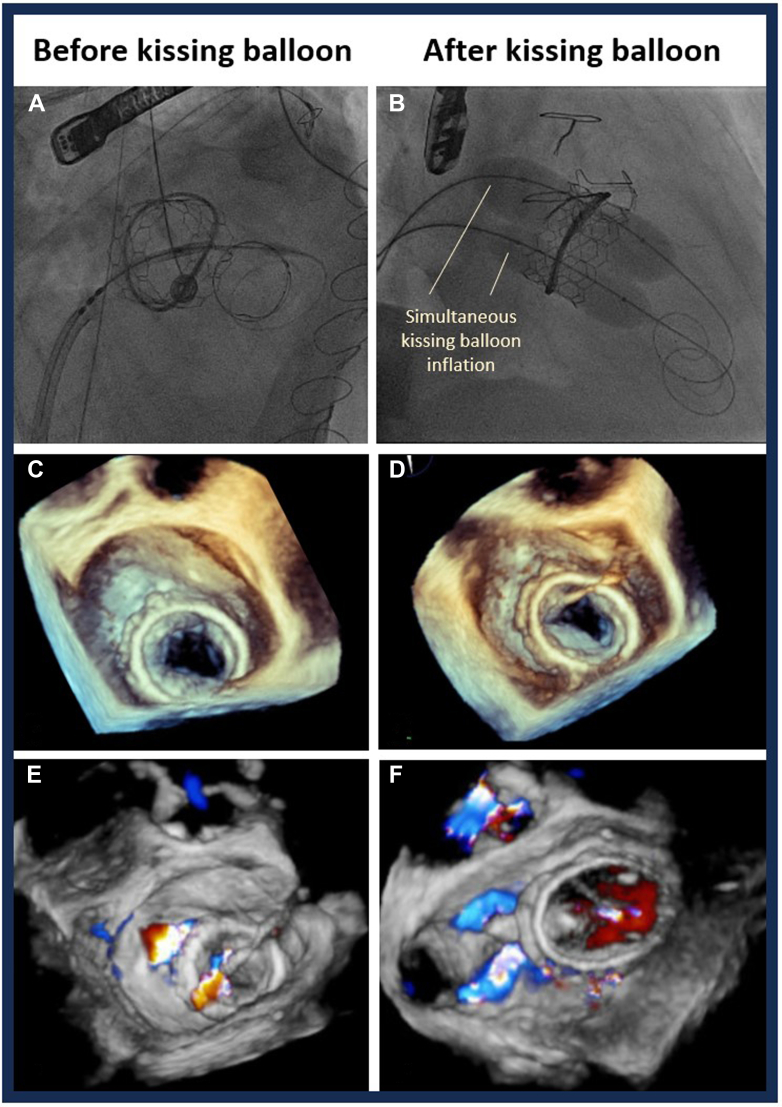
The Effect of Kissing Balloon Dilatation on THV Appearance Appearance of the transcatheter heart valve before (A, C, E) and after (B, D, F) kissing balloon dilatation in the angiographic (A and B) and 3-dimensional transesophageal echocardiography views (C to F) with (E and F) and without (C and D) color Doppler. (D and F) A significantly more elliptical shape compared with the more circularized appearance seen in C and E.
